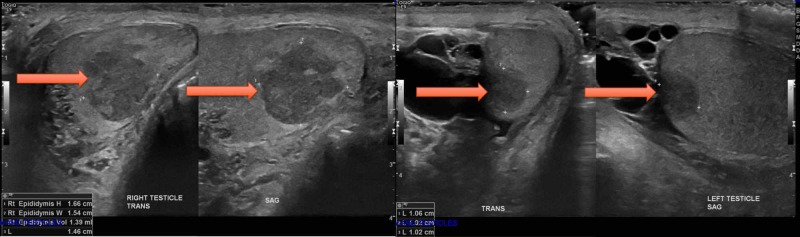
Ultrasound images of bilateral testicles The right testicle has a 1.6 x 1.5 x 1.4 cm vascular mass and 2.8 x 2.2 x 1.9 cm epididymal cyst. The left testicle has a 1.1 x 1.0 x 1.0 cm vascular hypoechoic mass and 4.3 x 3.4 x 2.6 cm epididmal cyst.
